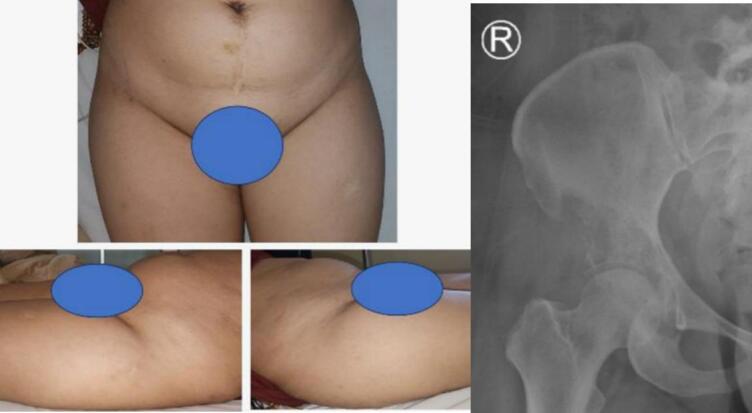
Clinical appearance at pelvic region (left). Pre-surgical radiographs (right).
